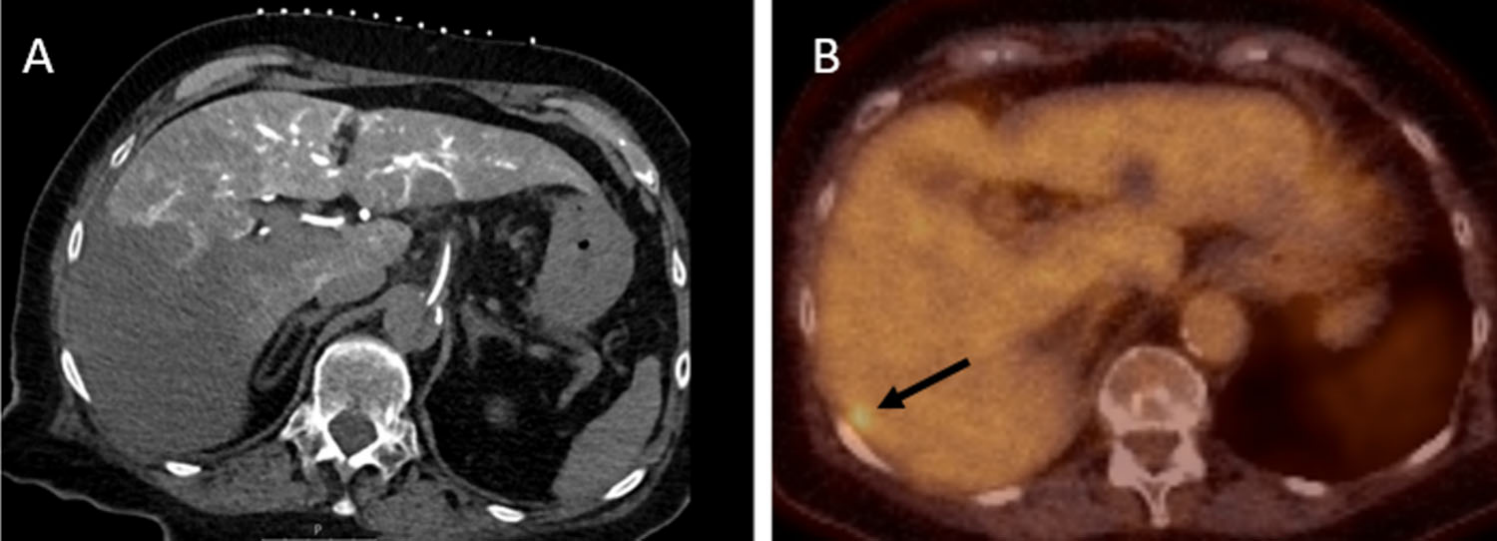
Case with aberrant anatomy: aberrant right hepatic artery from the superior mesenteric artery. This case presents a patient with an aberrant right hepatic artery originating from the superior mesenteric artery, resulting in a CTHA (catheter in the common hepatic artery originating from the celiac trunk) of which a large part of the right hepatic lobe could not be assessed. The follow-up 18F-FDG PET-CT reveals a subcapsular CRLM in segment VII that was subsequently invisible on CTHA, due to the segmented vascular supply
